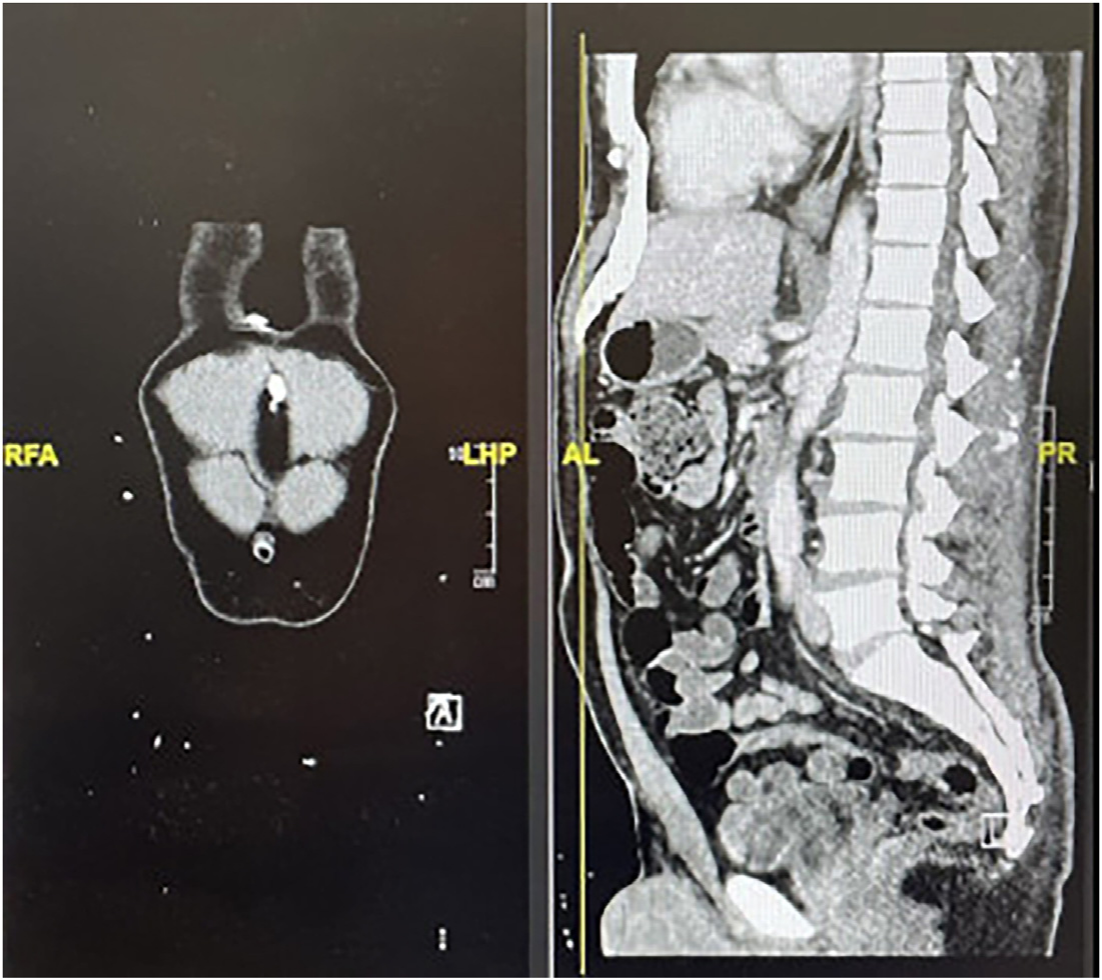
Preoperative computed tomography scan. (AL, anterior left; LHP, left head posterior; PR, posterior right; RFA, right foot anterior.)
